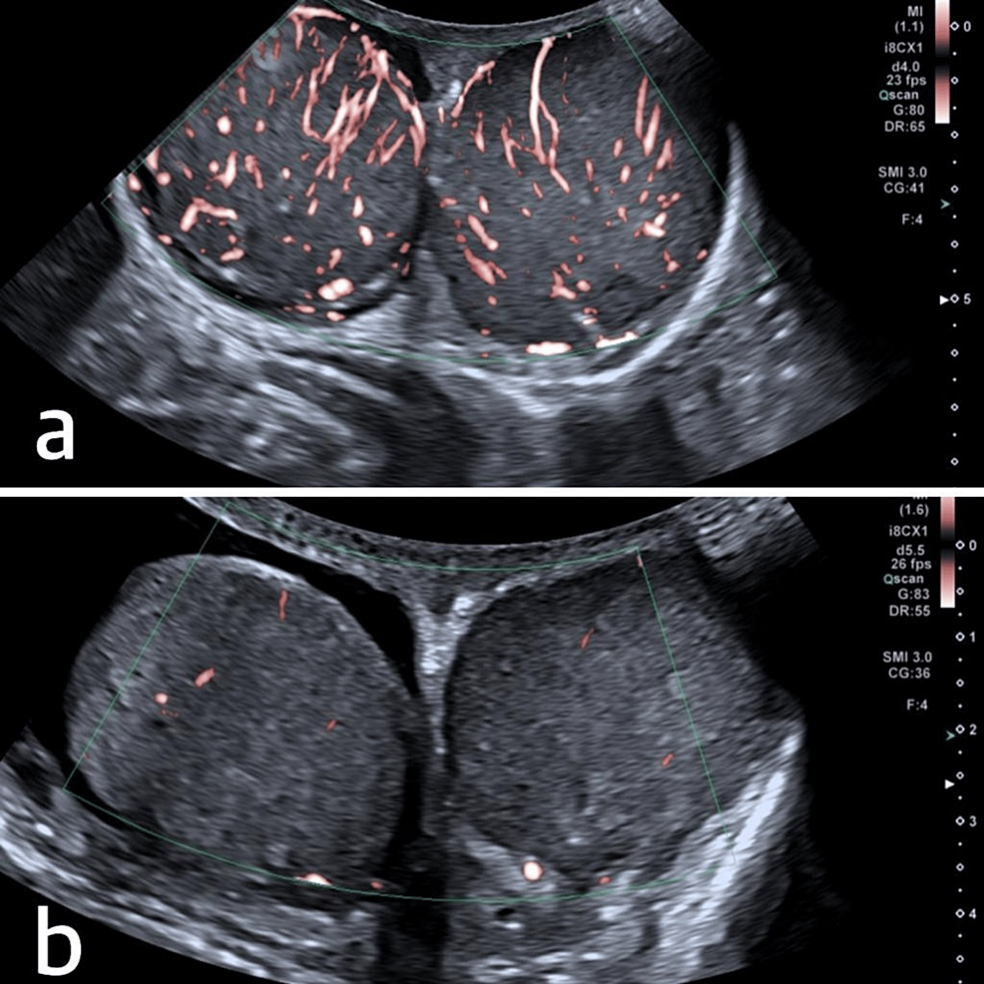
(a) Scrotal DUS image showing heterogeneity in the bilateral testicles with abnormally increased vascularity. (b) Post-treatment scrotal DUS image demonstrating the regression of vascularity in the bilateral testicles. DUS, Doppler ultrasound
